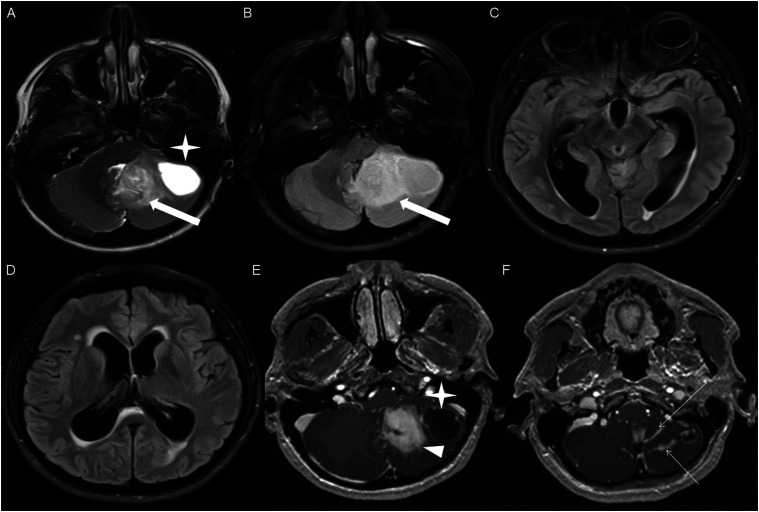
Axial T2 weighted image (A), axial FLAIR (B-D) and axial post-contrast T1 weighted image (E-F), show a T2/FLAIR hyperintense mass involving the left cerebellum (A-B, thick white arrow), with mixed cystic (A, star) and solid enhancing components (E, arrowhead). Inferiorly, there is surrounding spiculated, finger-like enhancement (F, thin arrows). There is mass effect and effacement of the fourth ventricle, with obstructive hydrocephalus and transependymal CSF flow.
